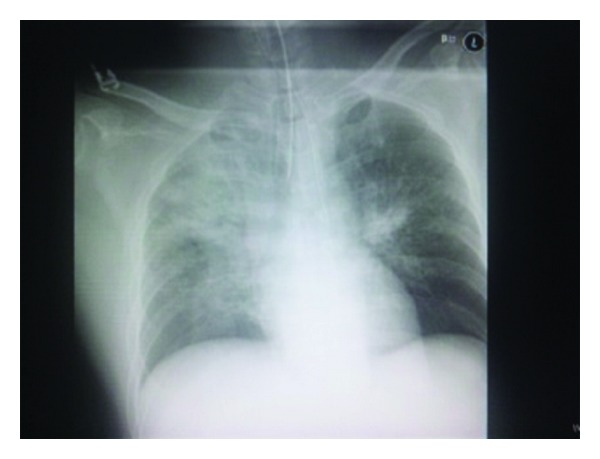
Chest roentgenogram after reintubation is shown. Marked shadow of the butterfly is seen.
